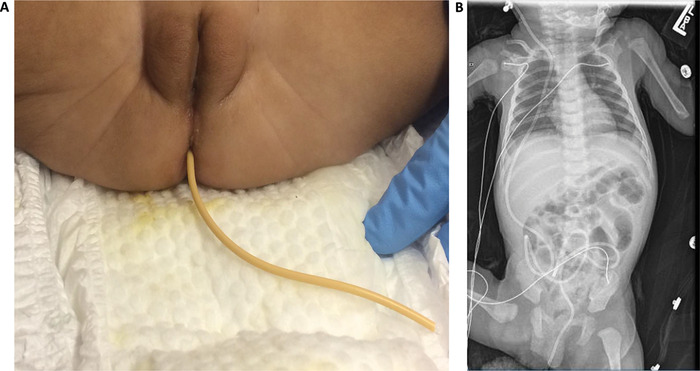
(A) Transanal ventriculoperitoneal (VP) shunt extrusion from the patient. (B) Radiographic image demonstrating VP shunt continuity.
